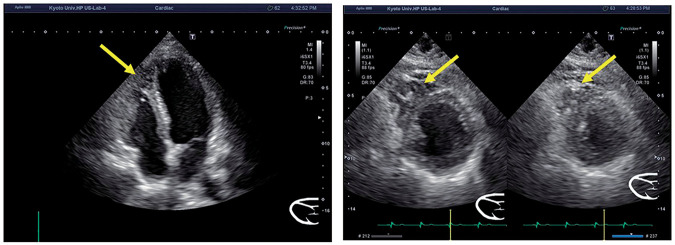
Echocardiography revealed an occupying lesion with a cystic appearance at the apex and within the ventricular septum (cardiac tumor: yellow arrows).
